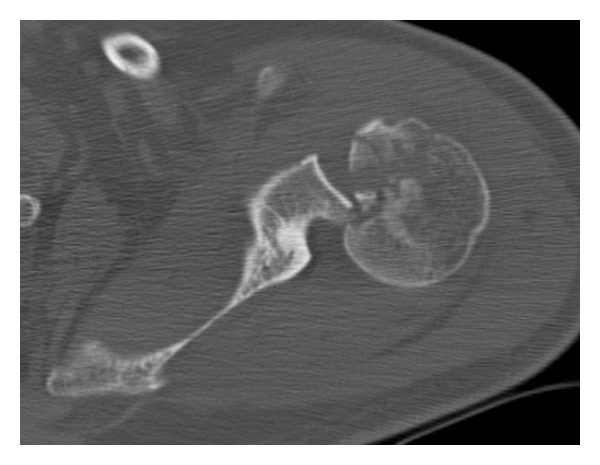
CT scan underlines the loss of cartilaginous surface and the posterior dislocation.
